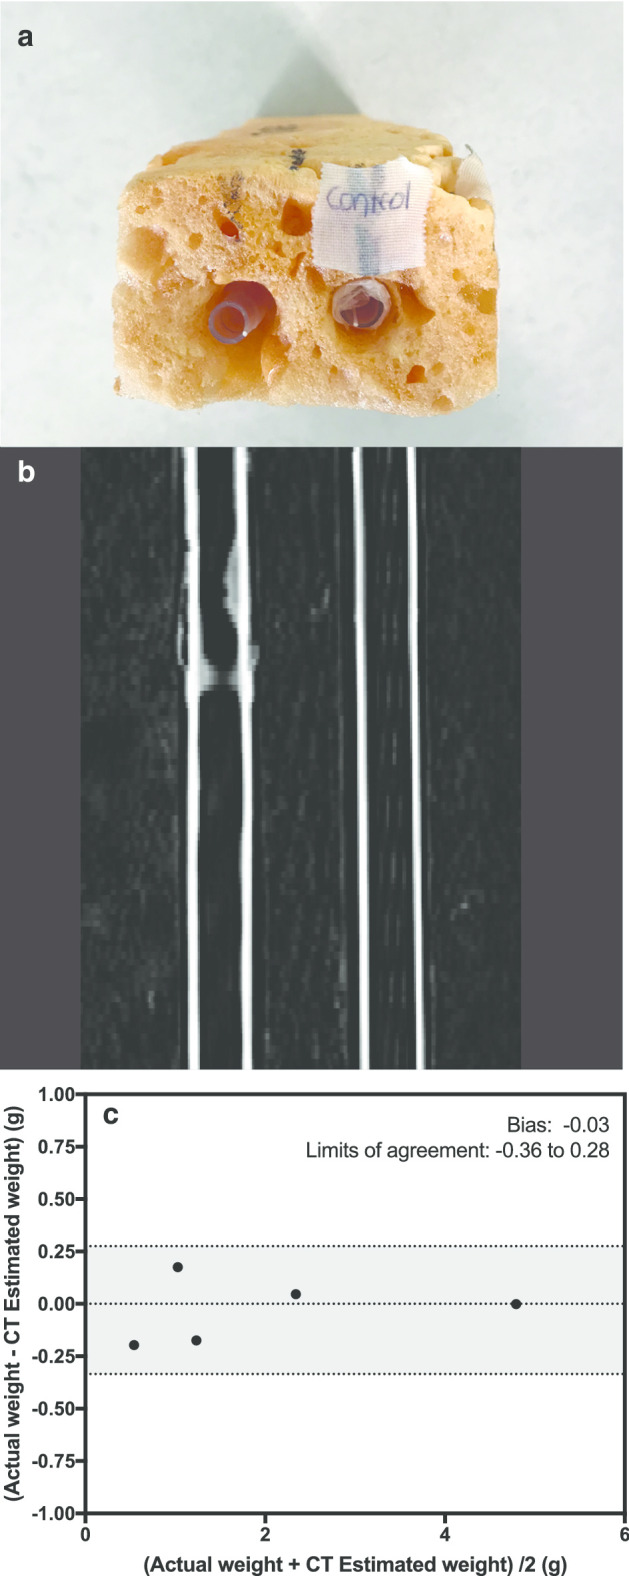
Tube mold used for image acquisition (a) and corresponding computed tomography image (b) of a partially obstructed tube (left) and the control tube (right). Bland–Altman analysis between CT-estimated and scale-measured mucus weight using a threshold of − 770 HU (c). CT, computed tomography.
